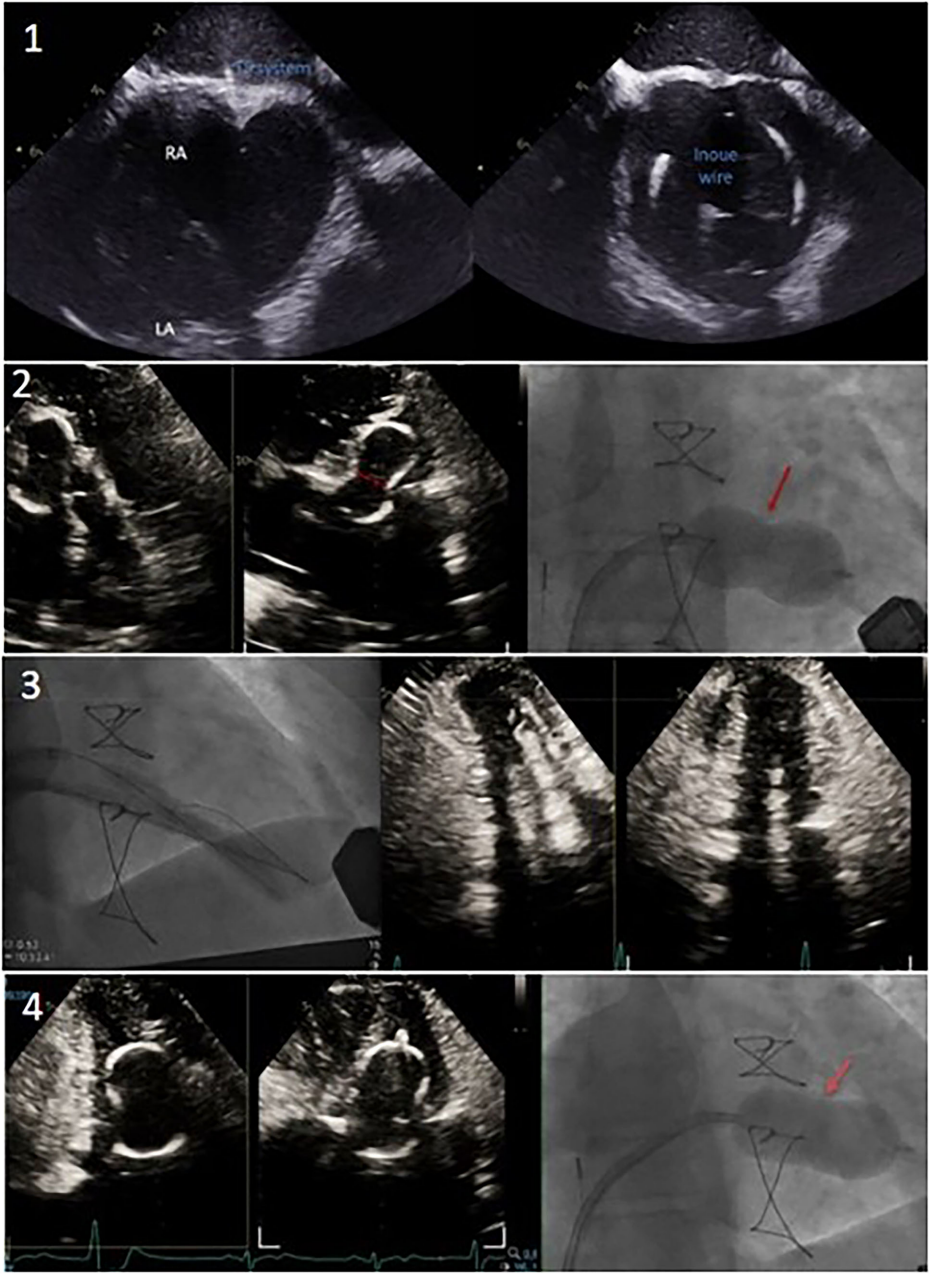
Step‐by‐step procedure. Step 1: Intracardiac echocardiogram ICE‐guided transseptal puncture, septal dilation, and delivery of a 28 mm Inoue balloon. Step 2: Multiple inflations with the 28 mm Inoue balloon, with failure to split the commissures. Step 3: Upsizing access to 22 Fr and delivering two 8.5 Fr steerable sheaths into the left atrium (LA) for simultaneous inflation of two 8.5 × 60 mm TMVL shockwave balloons. Step 4: Reinflation with the 28 mm Inoue balloon following TMVL treatment, achieving good balloon expansion. [Color figure can be viewed at wileyonlinelibrary.com]
